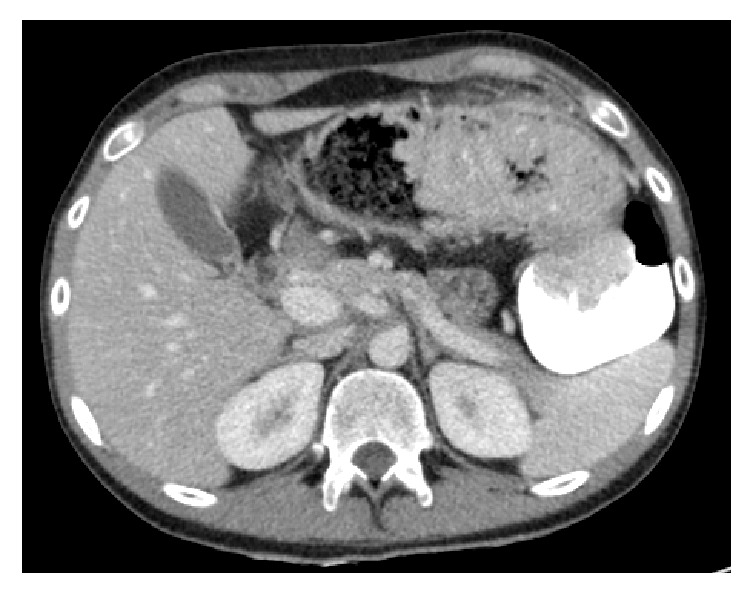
Abdominal CT scan showing obstructive GIP of the splenic flexure.
